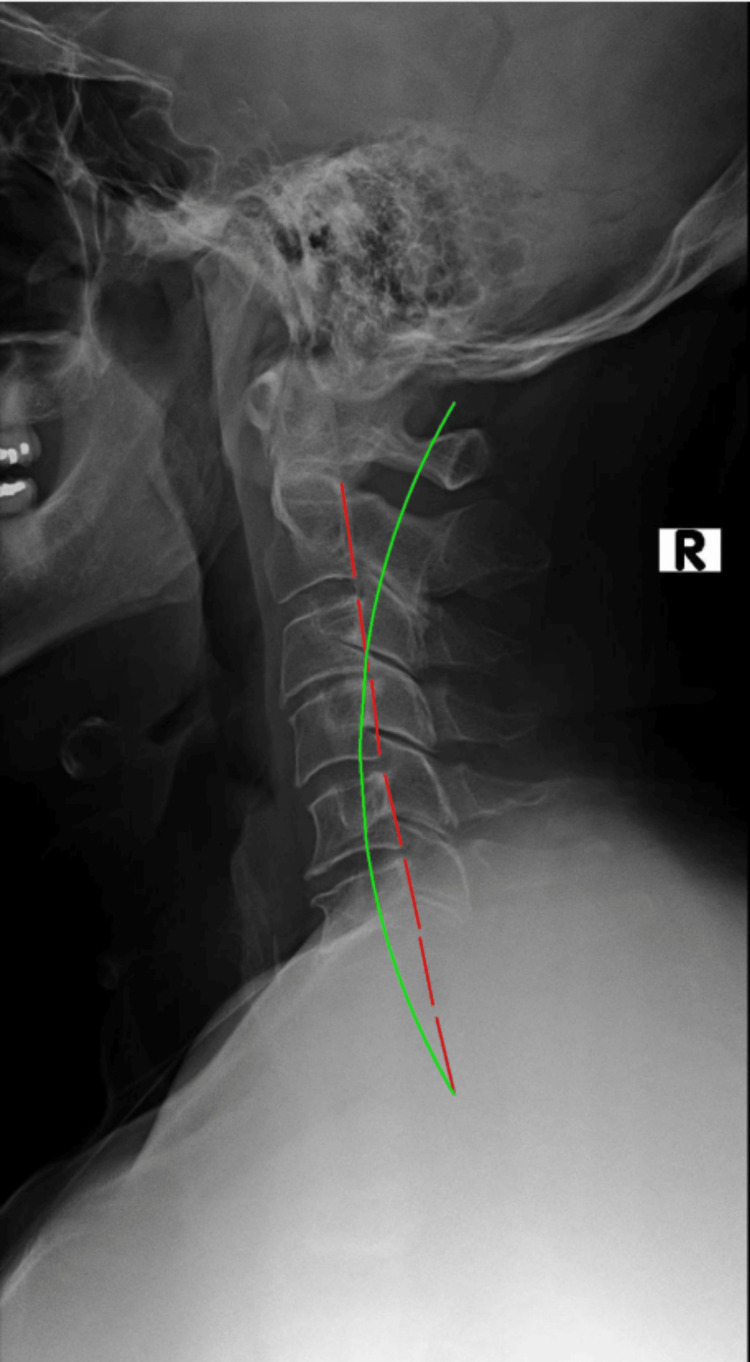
Re-examination lateral cervical radiograph following 12 in office treatments and 24 Denneroll® home treatments The red line demonstrates abnormal spine alignment. The green line represents ideal lateral cervical lordosis.
